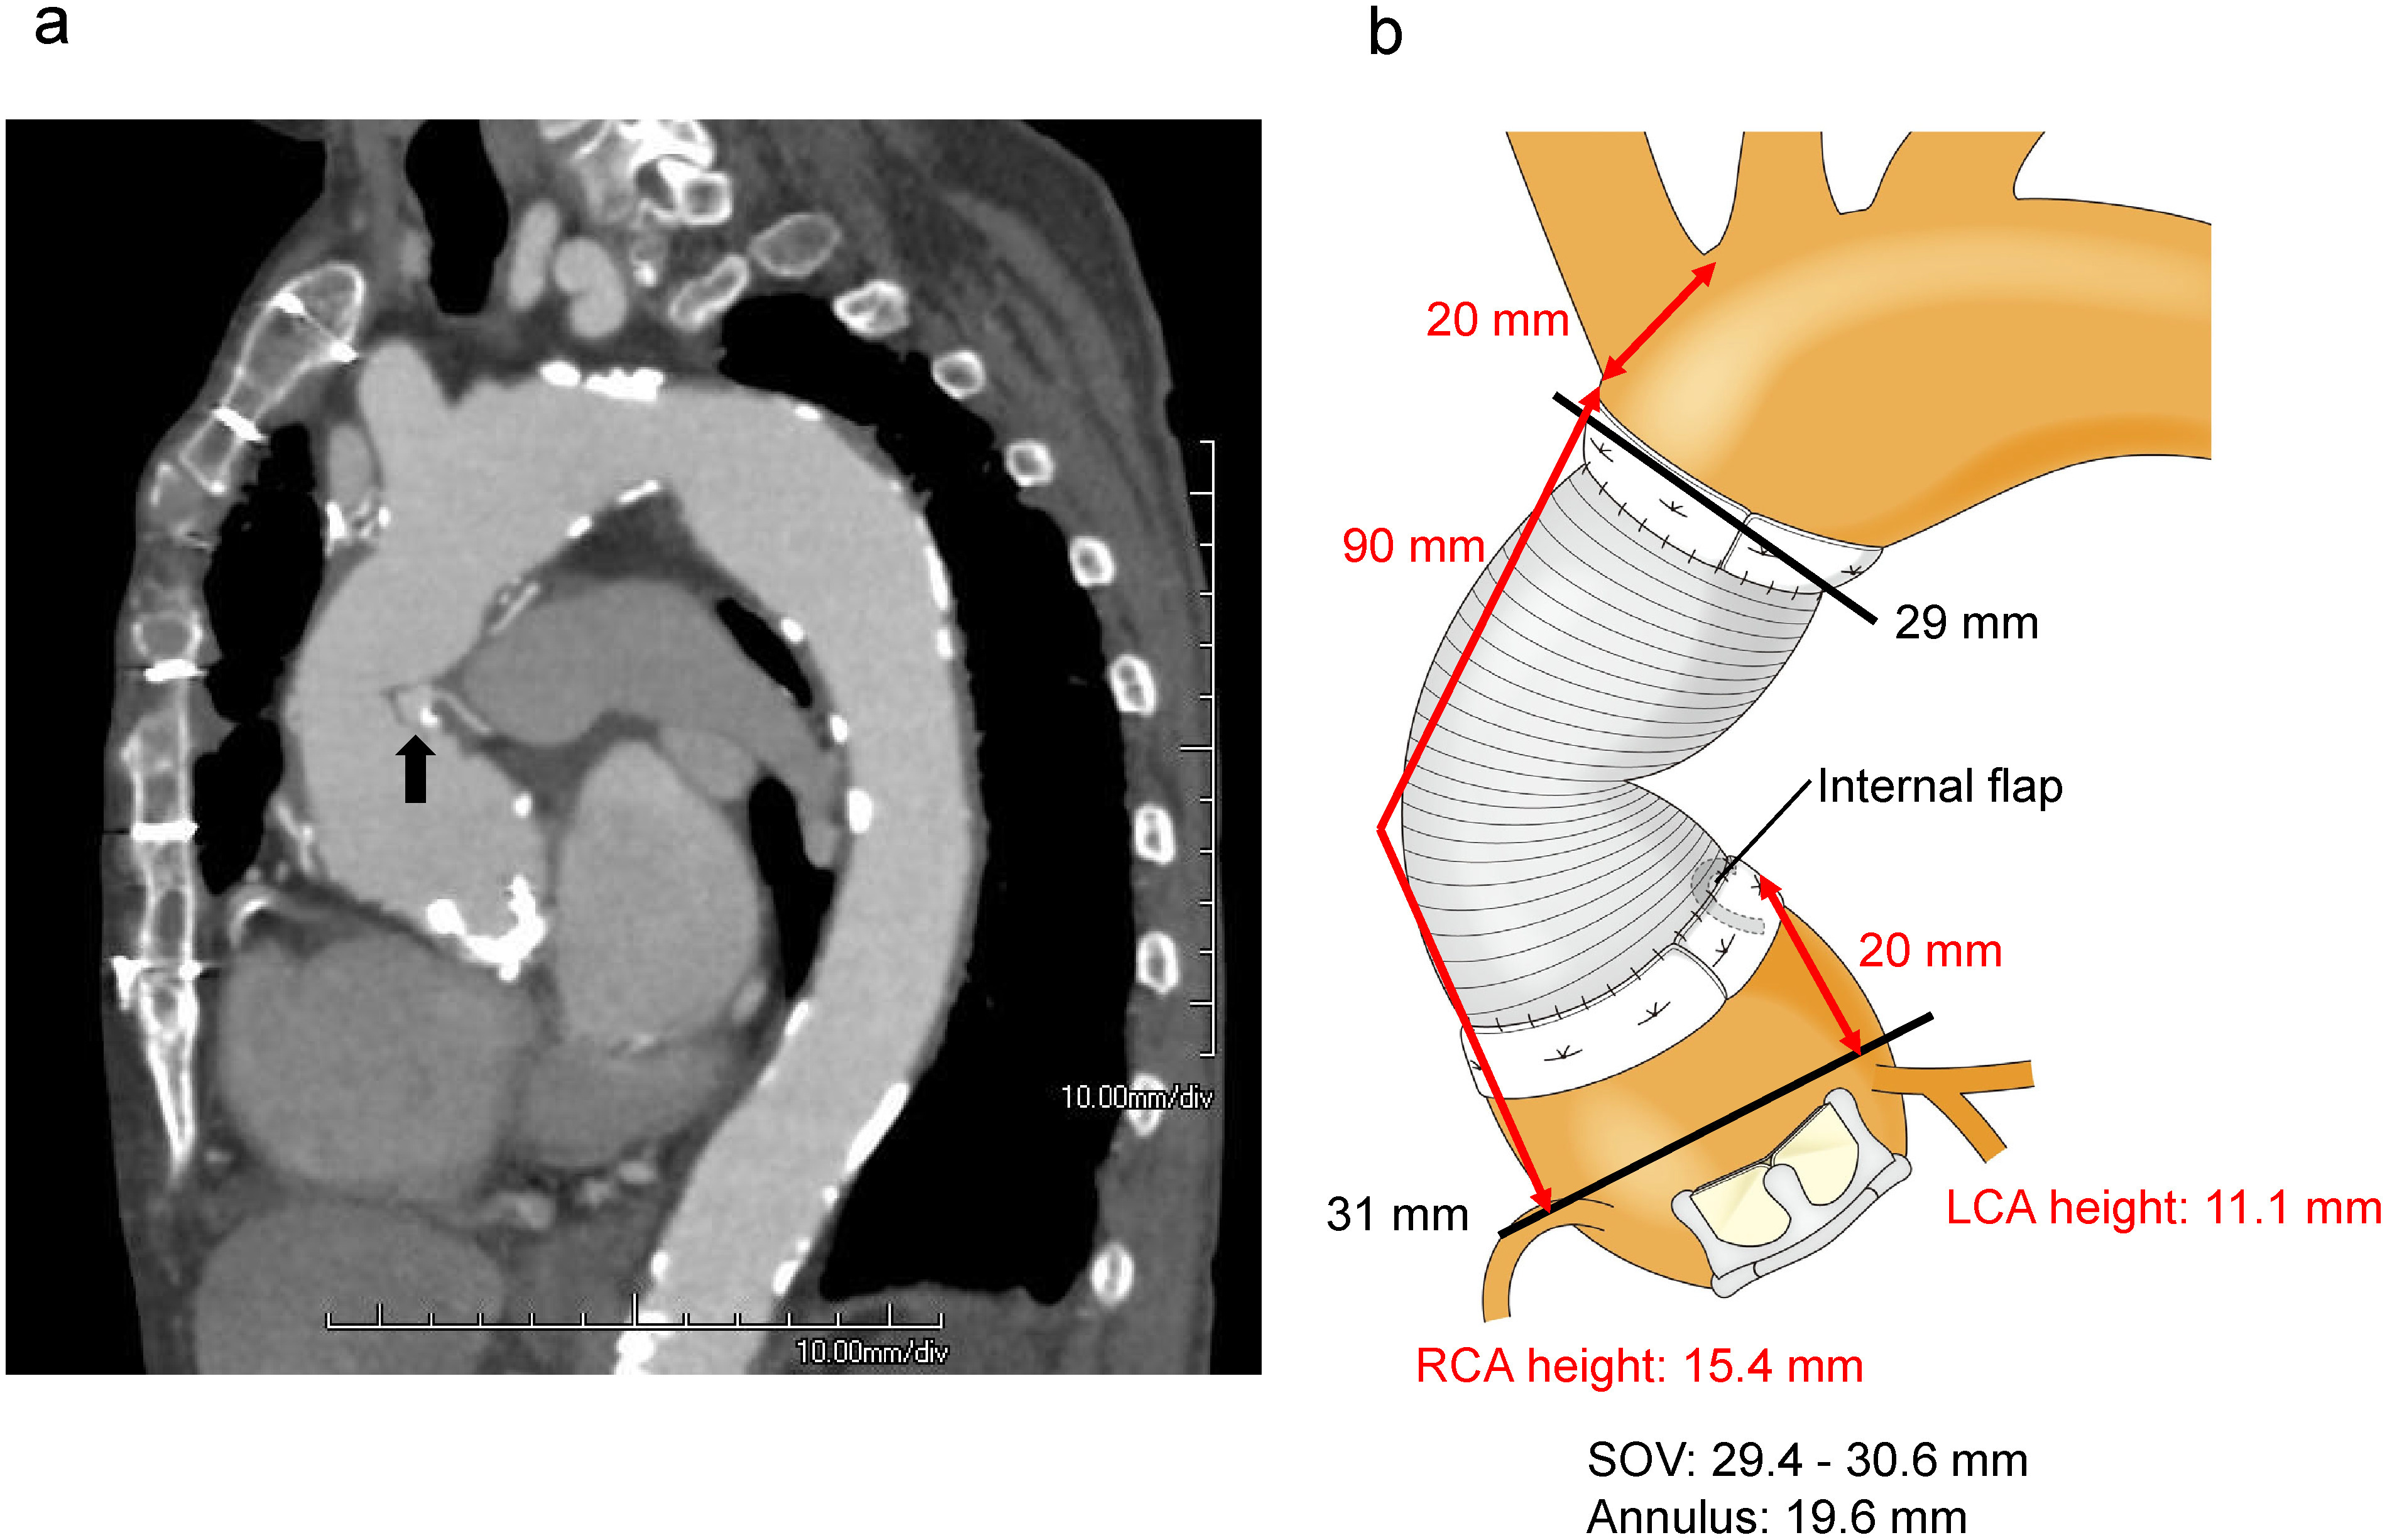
Fig. 1 Computed tomography showing (a) a flap (arrow) at the proximal anastomosis site of the ascending aorta, and (b) a scheme showing the measurement values of the aortic root complex, ascending aorta, and brachiocephalic artery.
